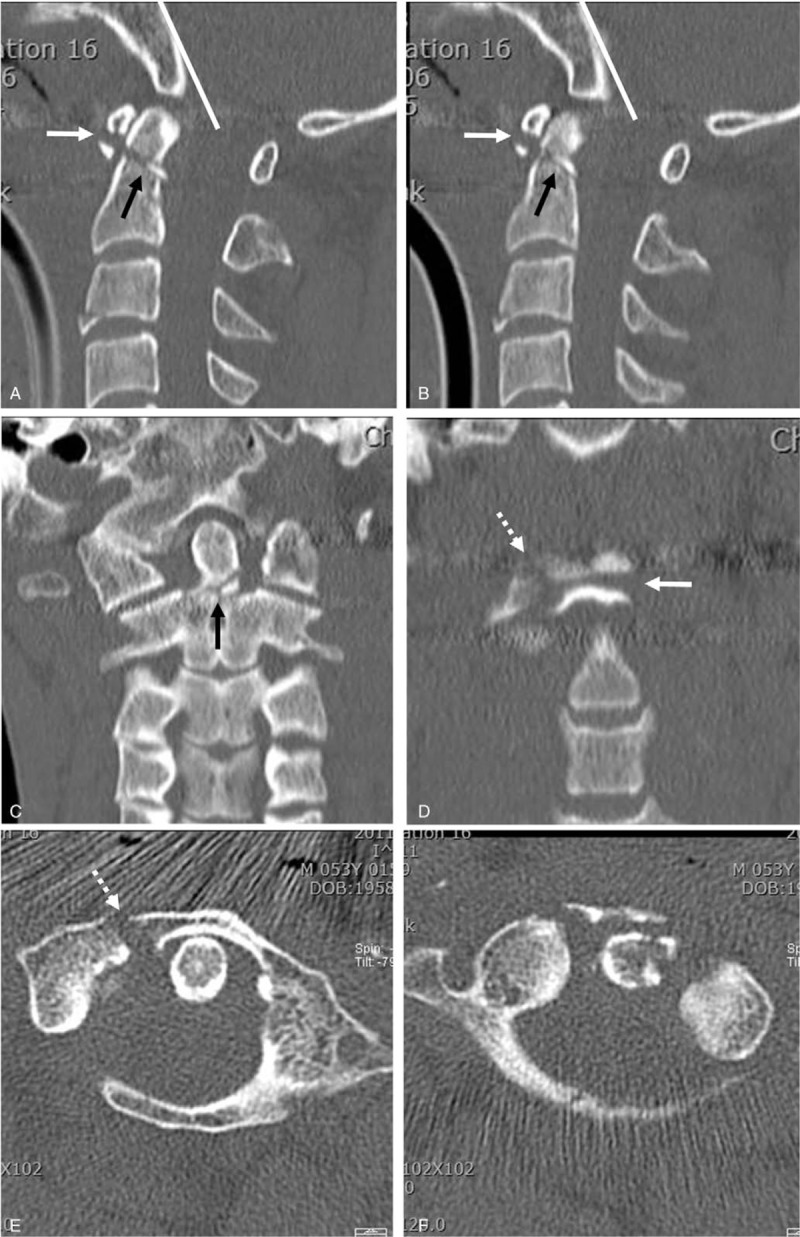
Sagittal (A and B) reconstructed CT scans showing running of the Wackenheim line (white line) behind the dens, indicating posterior atlantooccipital dislocation, dens type II fracture with comminution (Anderson - D’Alonzo classification) (black arrow), and fracture of the C1 anterior arch. Coronal (C and D) reconstructed CT scans revealed dens type II fracture with comminution and widening of the right atlantodental interval. Axial (E and F) CT scans showed a sagittal split fracture of C1 (white dot arrow) with a small avulsion fragment from a right lateral mass of C1, suggestive of a type II transverse atlantal ligament injury. CT = computed tomography.
